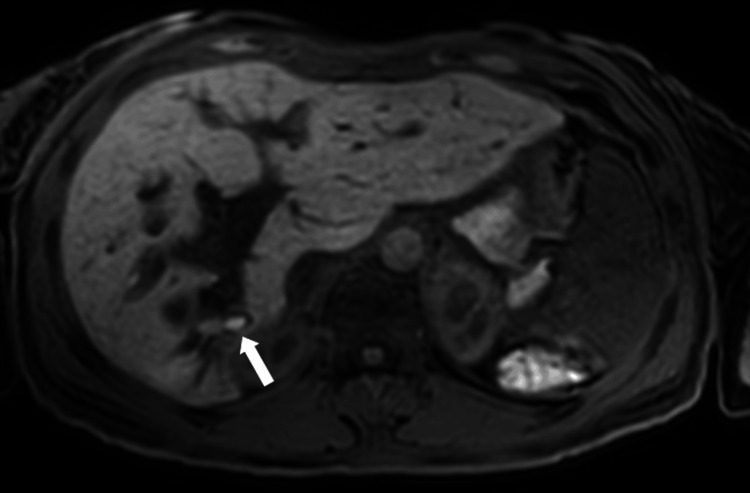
Axial T1-weighted MRI of the liver Axial T1-weighted MRI demonstrating biliary casts within the bile ducts, appearing hyperintense (white arrow).
